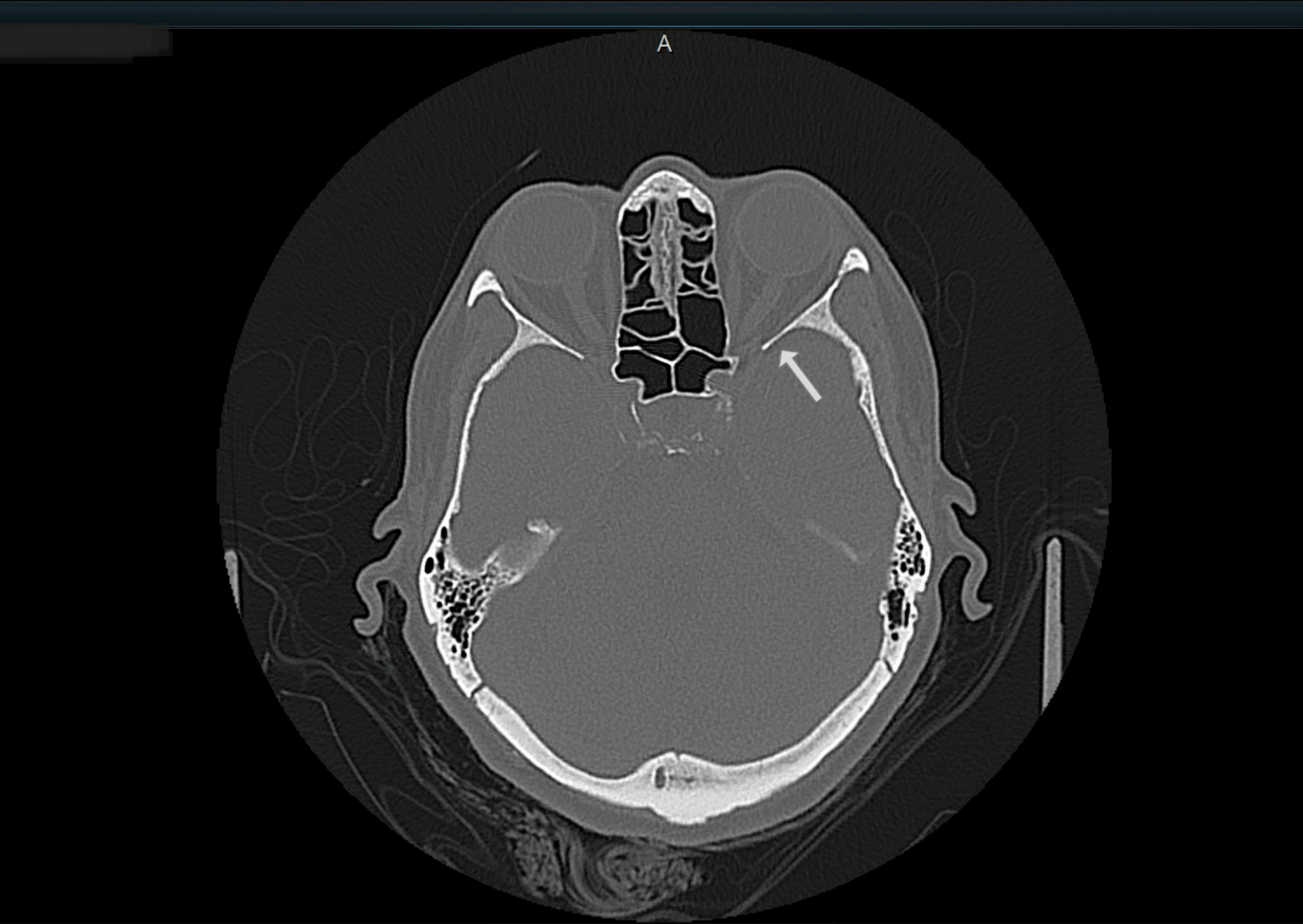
A CT-scan to see any presence of masses in the orbital cavity Checking for any masses present in the left orbital cavity
